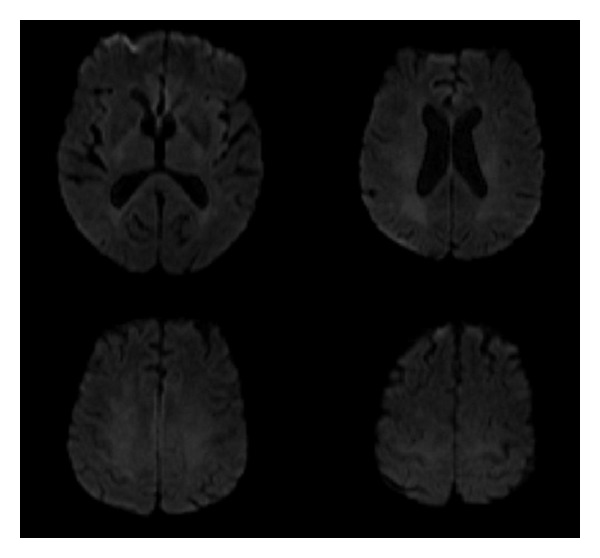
MRI of brain was normal; there is no sign of ischemic or tumor lesion.
